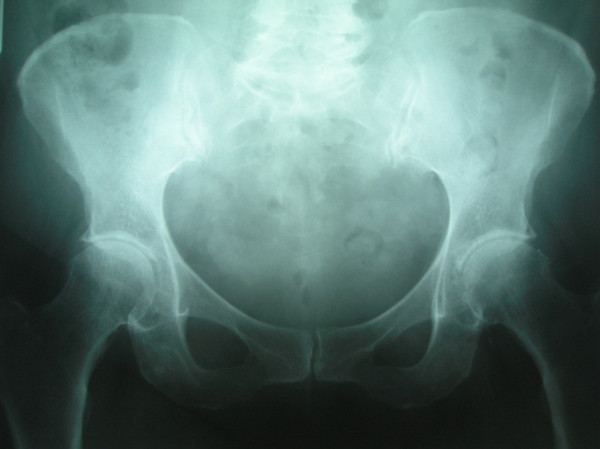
Anteroposterior radiographs obtained in a 70-year-old woman with painful bilateral hips of 4 months duration.
